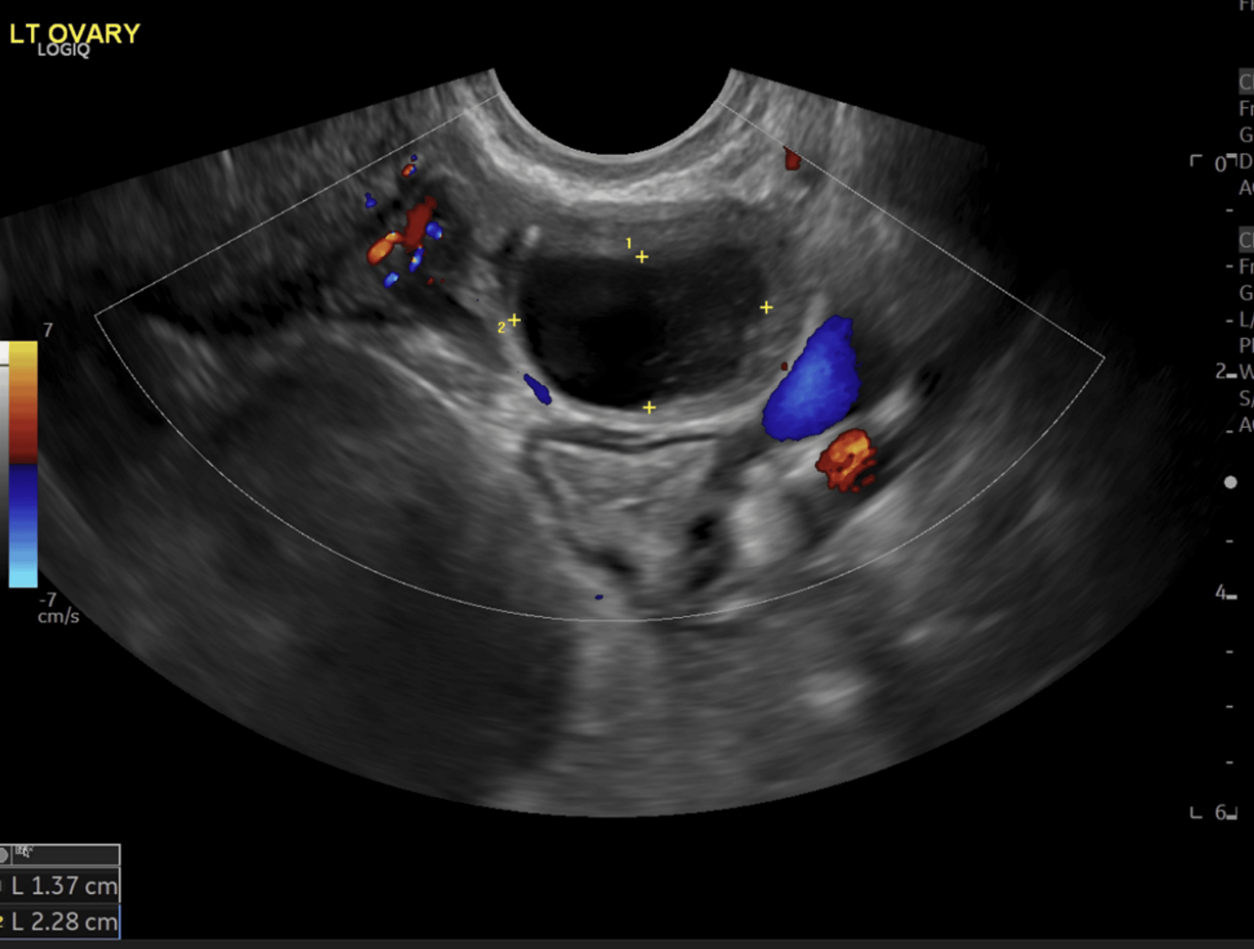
Transvaginal ultrasound with Doppler showing a haemorrhagic cyst in the left ovary
